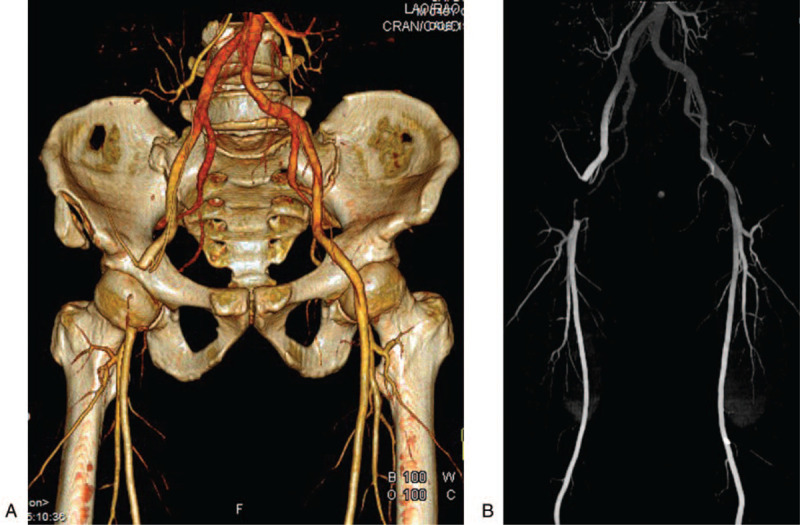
Case 1, CTA showed the right ilium wing fracture and a total occlusion of the distal end of the right external iliac artery. CTA = computed tomography angiography.
